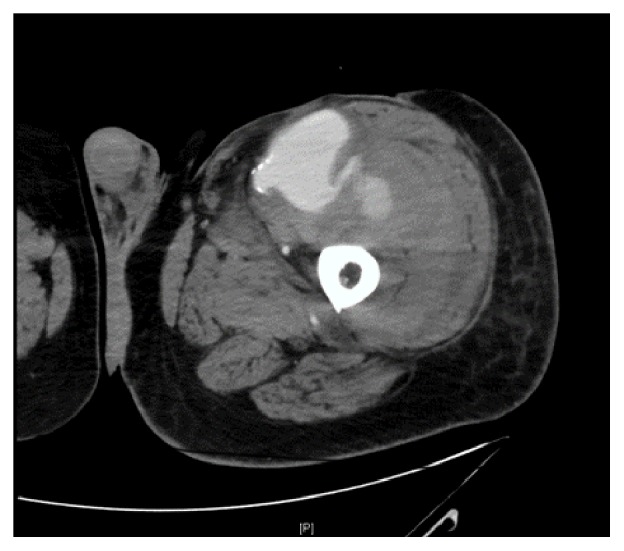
CTA of left lower extremity, axial images showing the anastomosis pseudoaneurysm with active extravasation.
